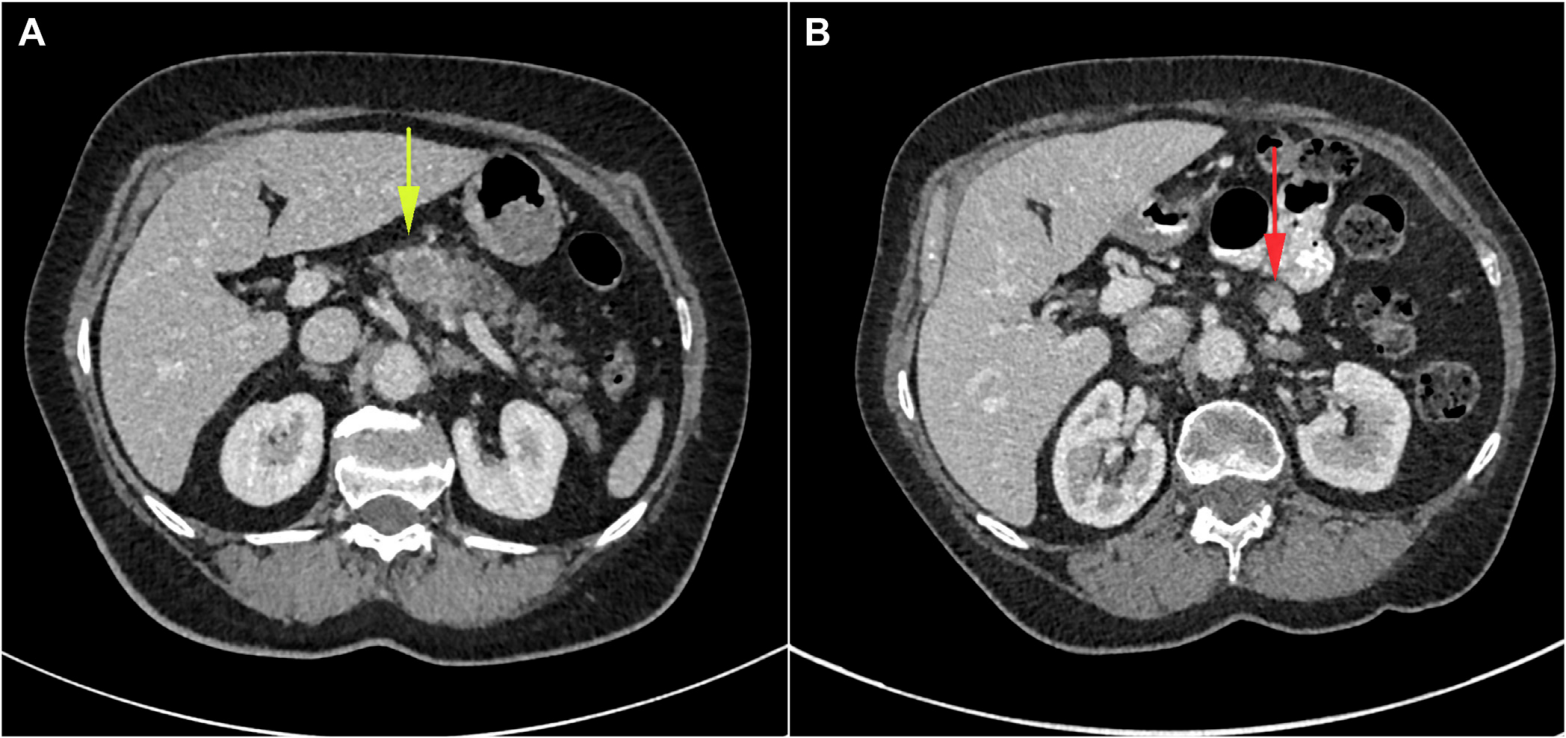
Contrast-enhanced computed tomography (CT) images of the pancreas before and after treatment with immune checkpoint inhibitor. (A) Yellow arrow: Pancreatic metastasis in the body of the pancreas measuring 2.4 cm in maximum axial diameter (baseline image). (B) Red arrow: Pancreatic atrophy and resolution of the metastasis (post-treatment image).
